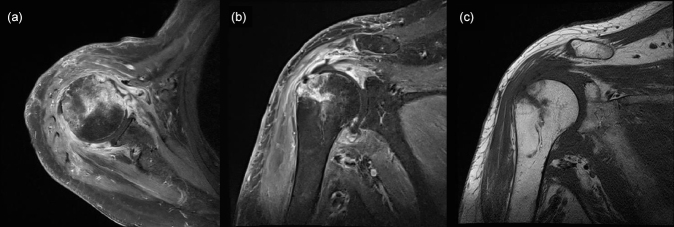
Magnetic resonance image obtained 3 weeks after surgery showing increased signal intensity in the cortex and medulla of the humeral head, while the same areas appear well-demarcated and darker in the non-enhanced image: (a) T1-weighted, contrast-enhanced fat suppression axial-view image; (b) T1-weighted, contrast-enhanced fat suppression coronal-view image; (c) T1-weighted, non-contrast-enhanced coronal-view image.
